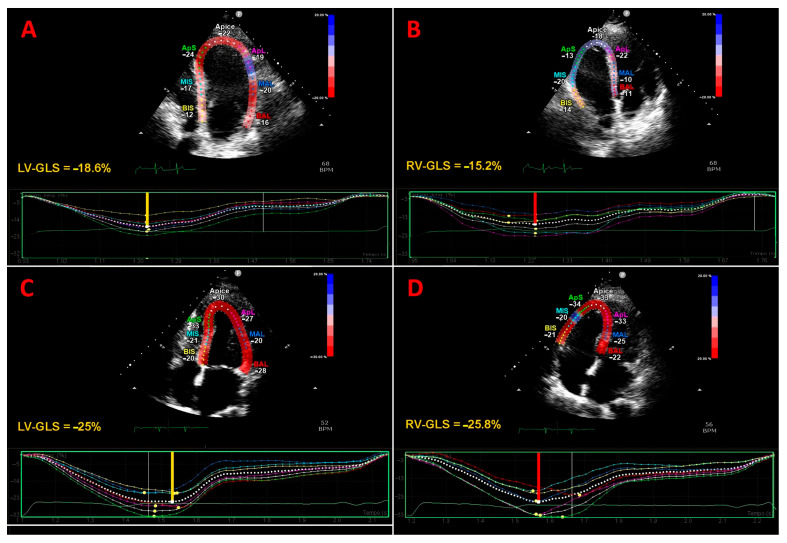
Representative examples of LV-GLS and RV-GLS assessments from the apical four-chamber view in a COPD patient (A,B) and in a healthy control without COPD (C,D). The bold yellow line and red line indicate the LV-GLS and RV-GLS magnitudes, respectively, obtained in the two individuals. COPD, chronic obstructive pulmonary disease; GLS, global longitudinal strain; LV, left ventricular; RV, right ventricular.
